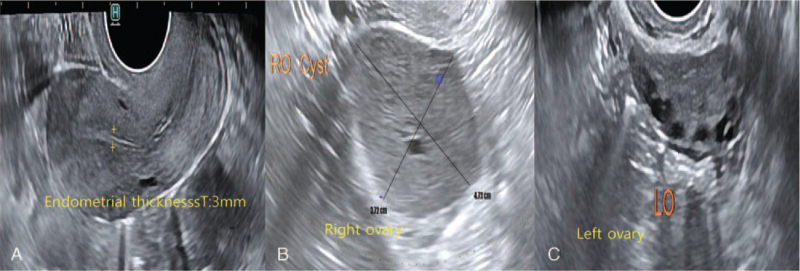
An ultrasound scan at admission. (A) The endometrial thickness was 3 mm. (B) An ultrasound scan revealed a 47 × 37-mm-sized round solid mass on the right ovary with a spongiform appearance and little vascularity. (C) The left ovary showed a PCO pattern.
